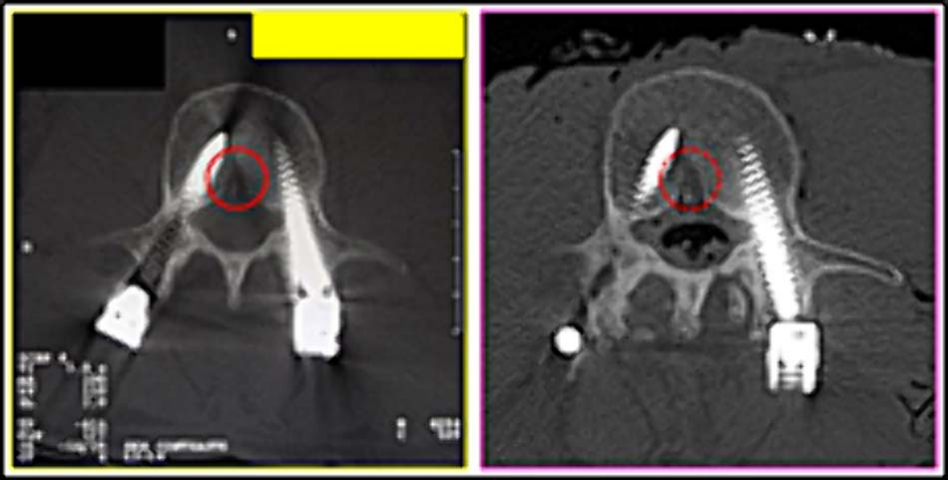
Posterior contour of the L3 vertebral body. Comparison between the axial slices of the antemortem (left) and postmortem (right) CT scans. Element of agreement.
